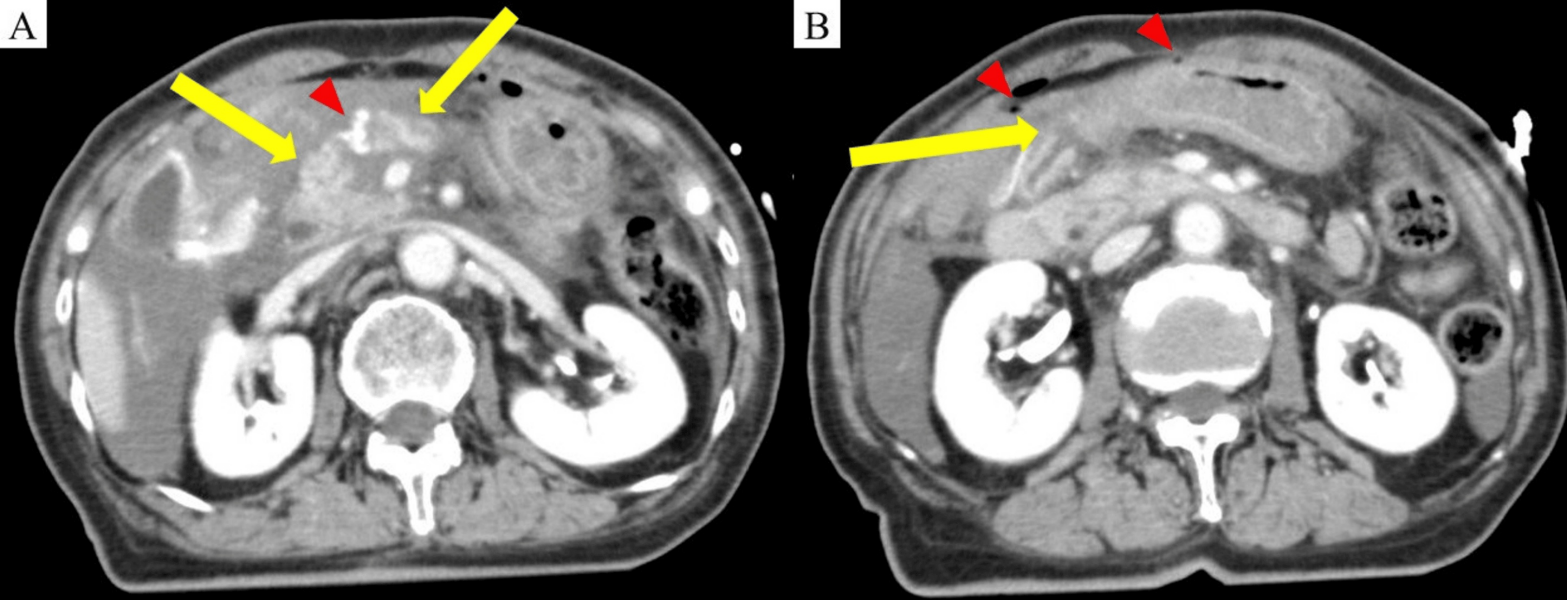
Contrast-enhanced abdominal computed tomography in the portal venous phase (coronal section) (A) A complete transection of the pancreas is observed just above the superior mesenteric vein (yellow arrow). Active bleeding from the distal right gastric artery was inferred (red triangle). (B) Disruption of mucosal continuity in the duodenal bulb is noted (yellow arrow). Additionally, free air is present in the abdominal cavity (red triangle).
